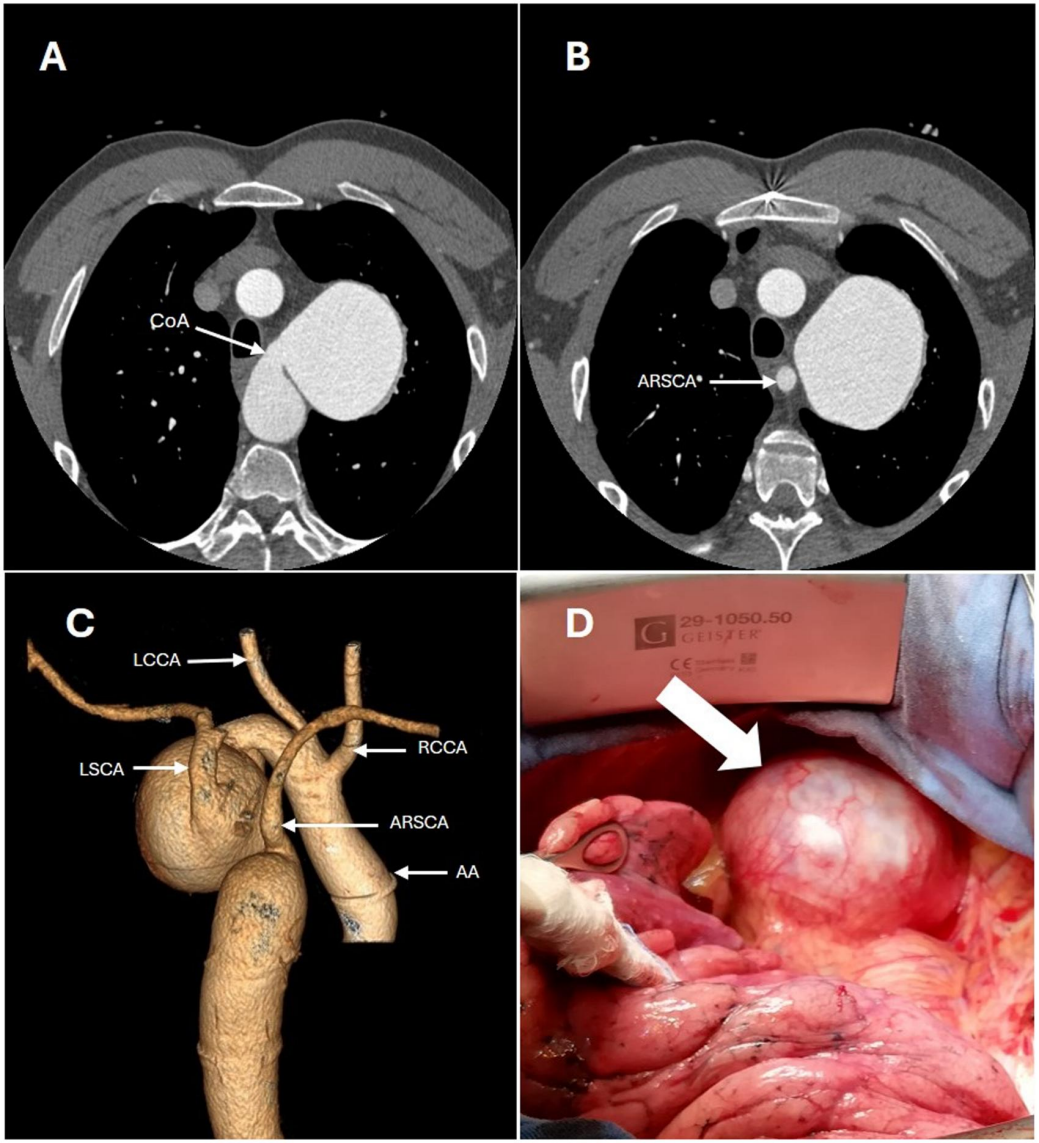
(A) Preoperative CT scan. (B) Pre-coarctation aneurysm with the arteria lusoria. (C) 3D reconstruction (the aorta is rotated 180°). (D) Intraoperative view of the pre-coarctation aneurysm through a left thoracotomy. AA: ascending aorta; ARSCA: aberrant right subclavian artery; CoA: coarctation of the aorta; LSCA: left subclavian artery; RSCA: right subclavian artery; Thick arrow: saccular aneurysm.
